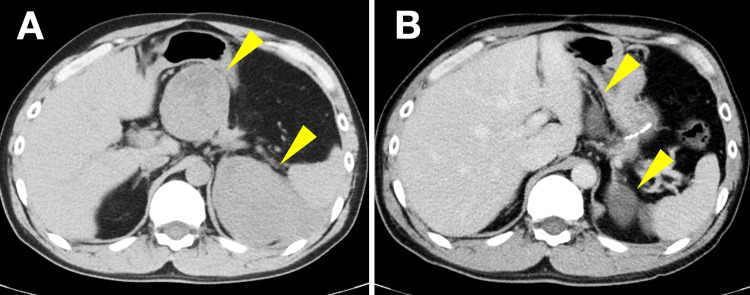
Surveillance CT demonstrating recurrent disease and treatment response (A) Image three years after imatinib discontinuation showing two recurrent lesions (arrowheads): 34 mm adjacent to the pancreatic body and 47 mm lateral to the spleen (sum: 81 mm). (B) Image after imatinib reintroduction demonstrating partial response per RECIST 1.1 criteria with lesion reduction to 14 mm and 18 mm (sum: 32 mm; 60.5% decrease) CT: computed tomography
